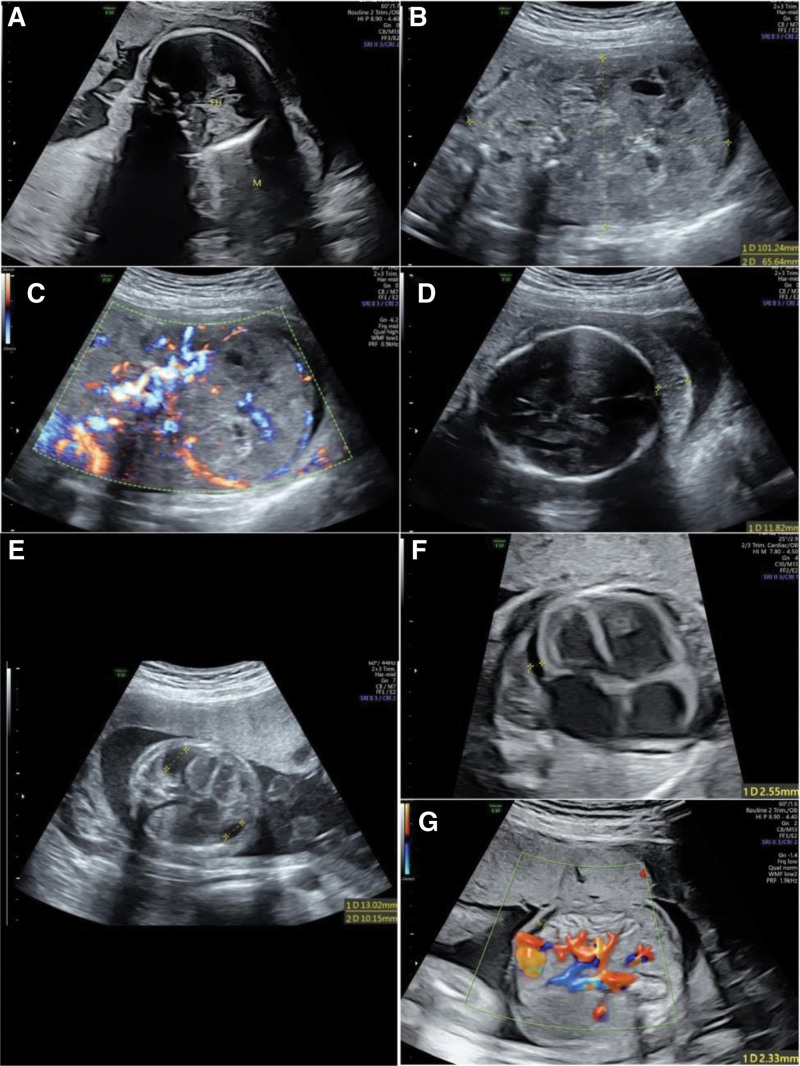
Targeted ultrasound sonogram. (A) Sagittal view of the mass in the head and neck. FH = fetal head, M = mass. (A–C) A heterogeneous solid mass measuring approximately 10.1 × 6.5 cm was observed in the head and neck, and rich blood flow signals were observed in the mass. (D) Fetal scalp edema, measuring approximately 1.1 cm wide. (E) Pleural effusion. The width of the left side measured approximately 1.3 cm and the width of the right side measured approximately 1.0 cm. (F) Pericardial effusion. It measured approximately 0.3 cm wide. (G) Fluid in the abdominal cavity. It measured approximately 0.2 cm wide.
